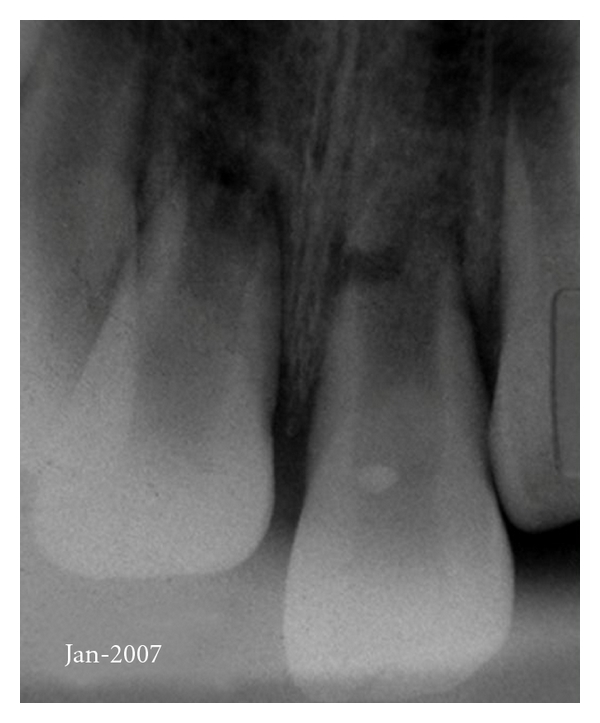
Preoperative radiograph. Severe intrusive luxation of tooth 11 and root fractures of apical thirds of both maxillary central incisors.
